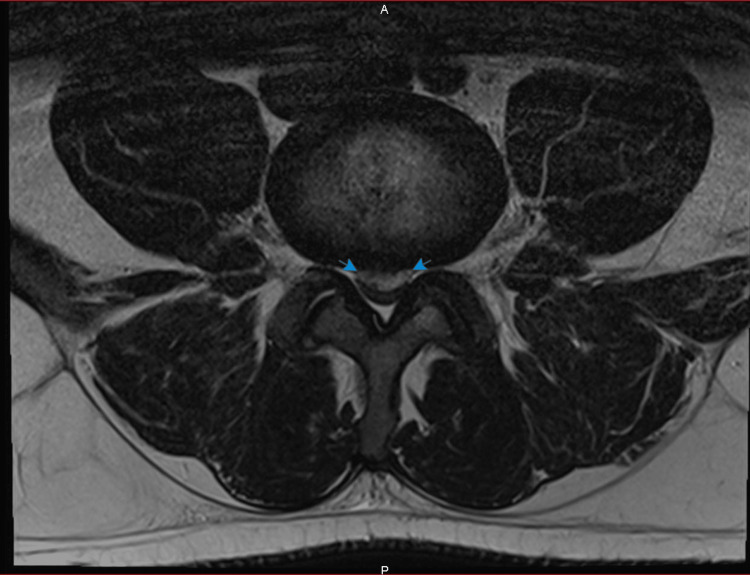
Axial T2-weighted image. Axial T2-weighted image showing the mass compressing the spinal cord at L4-L5 level. The structure is delineated by two blue arrows on each side.
